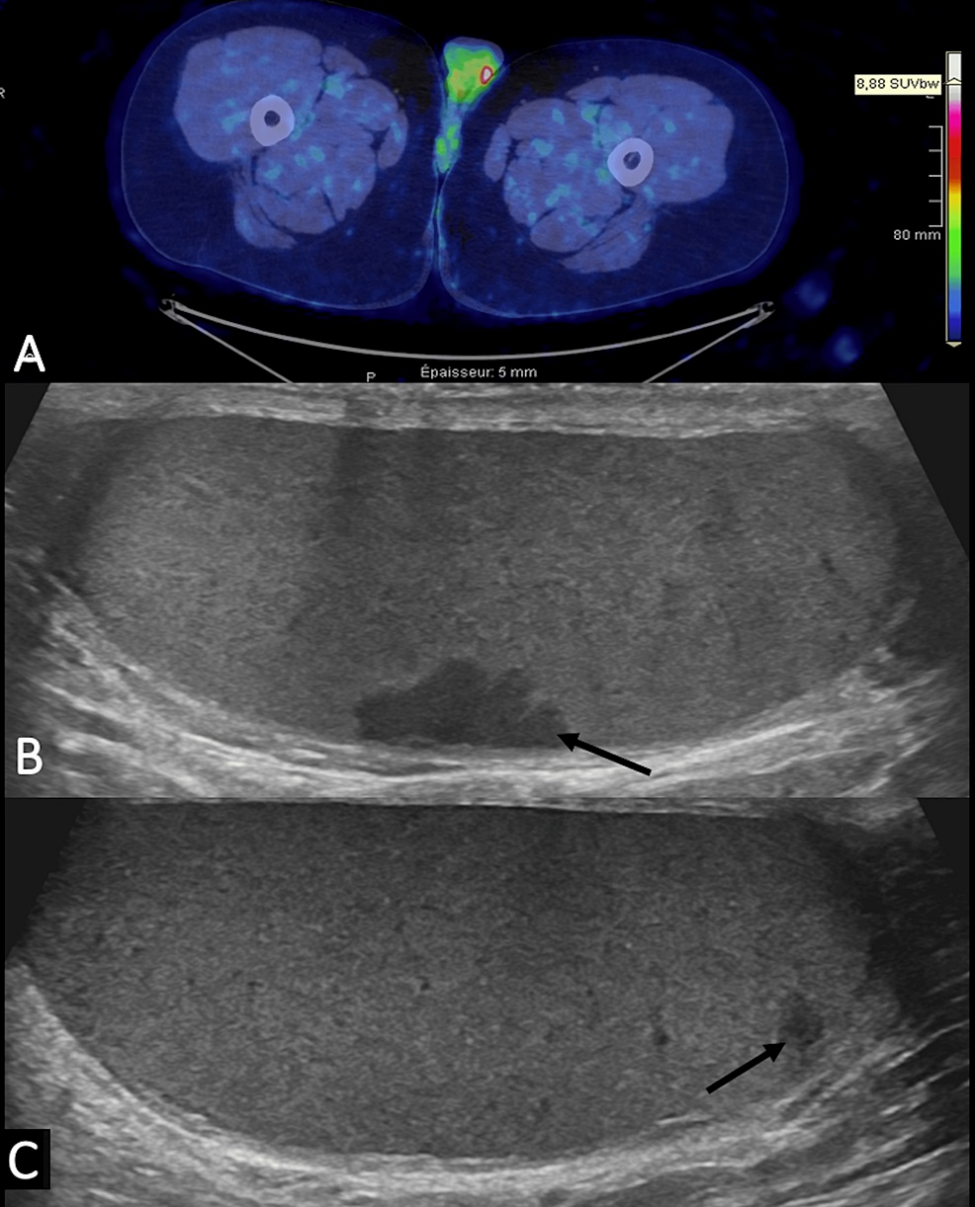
Axial FDG-PET/CT and scrotal ultrasonography Axial FDG-PET/CT (A) shows in white left testicular lesion with elevated SUV. Ultrasonography shows two nodular hypoechoic lesions (black arrows) in both testes appearing hypermetabolic on PET/CT, seen on the left (B) and the right (C). The SUVmax was 8.88. FDG-PET/CT: fluorodeoxyglucose-positron emission tomography/computed tomography, SUV: standardized uptake value, SUVmax: maximum standardized uptake value
